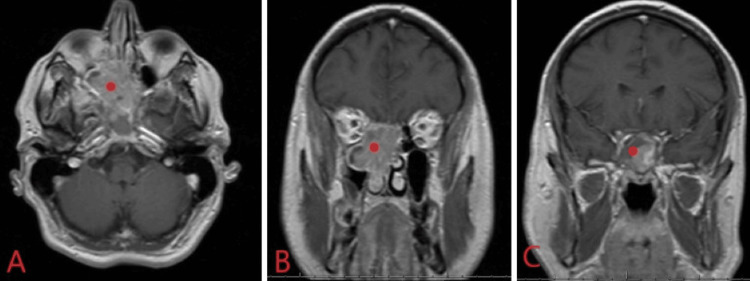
T1 with contrast MRI brain in axial (A) and coronal (B,C) planes showing an enhancing, expansile mass of the right nasal cavity (red dot) extending from the right ethmoid sinus to the bilateral sphenoid sinuses and right anterior skull base, with extension of the mass into the right orbital apex.
